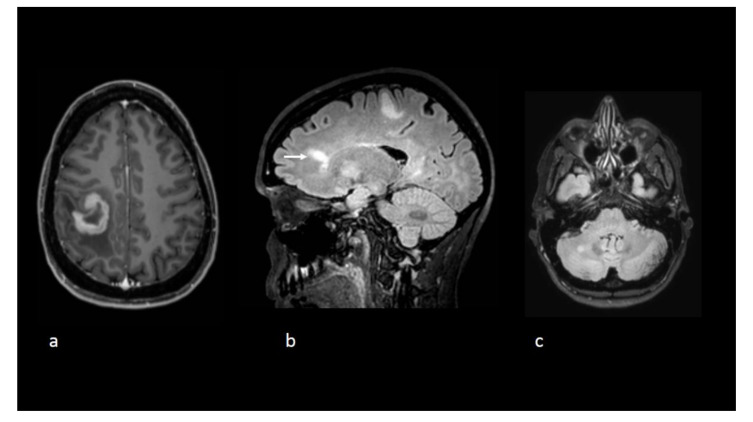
Axial T1-weighted postgadolinium image (a) reveals a tumefactive lesion centred on the central sulcus with a thick open-ring enhancement pattern before initiation of natalizumab. Sagittal (b) and axial (c). Fluid-attenuated inversion recovery (FLAIR) sequences show other nonenhancing lesions including one with a Dawson’s fingers appearance (white arrow).
